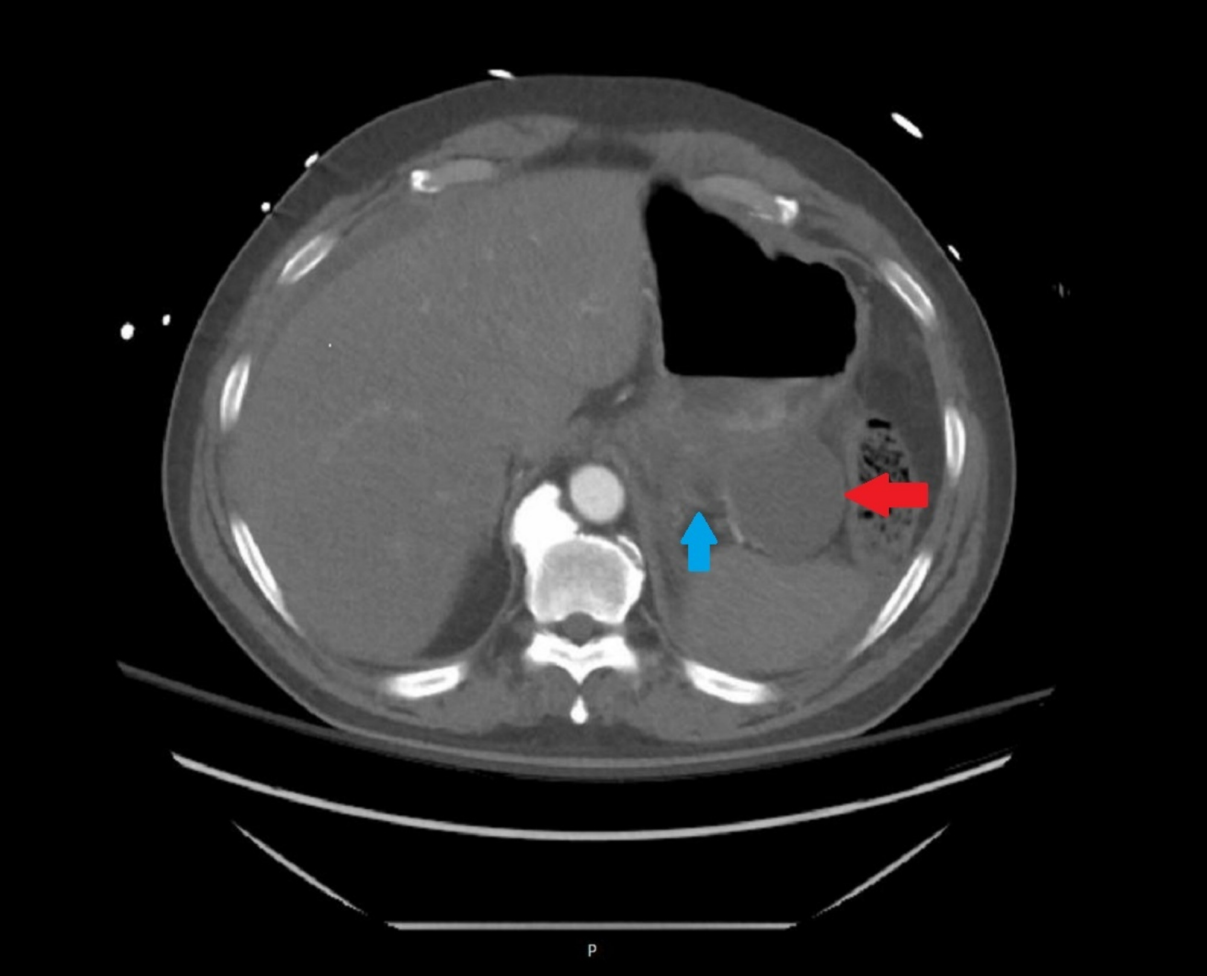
Case 2 CTA Abdomen and Pelvis Patient's CTA abdomen and pelvis showing pseudocyst (red arrow) and general pancreatitis (blue arrow). CTA: Computed tomography angiography
